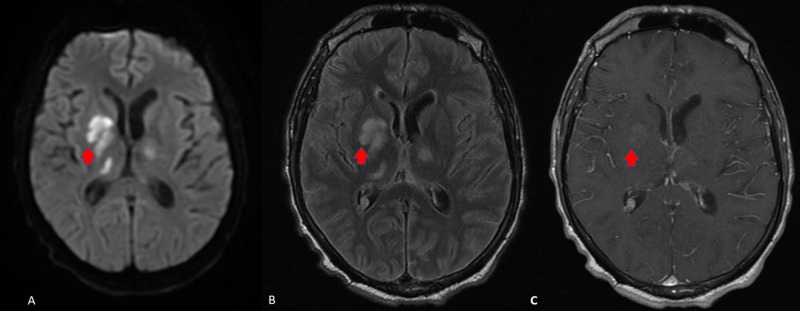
Brain magnetic resonance imaging (MRI) at presentation, prior to treatment with mirtazapine A) diffusion-weighted imaging (DWI); B) fluid-attenuated inversion recovery (FLAIR); C) contrast study. Lesion visible over deep gray matter of basal ganglion and thalami.
